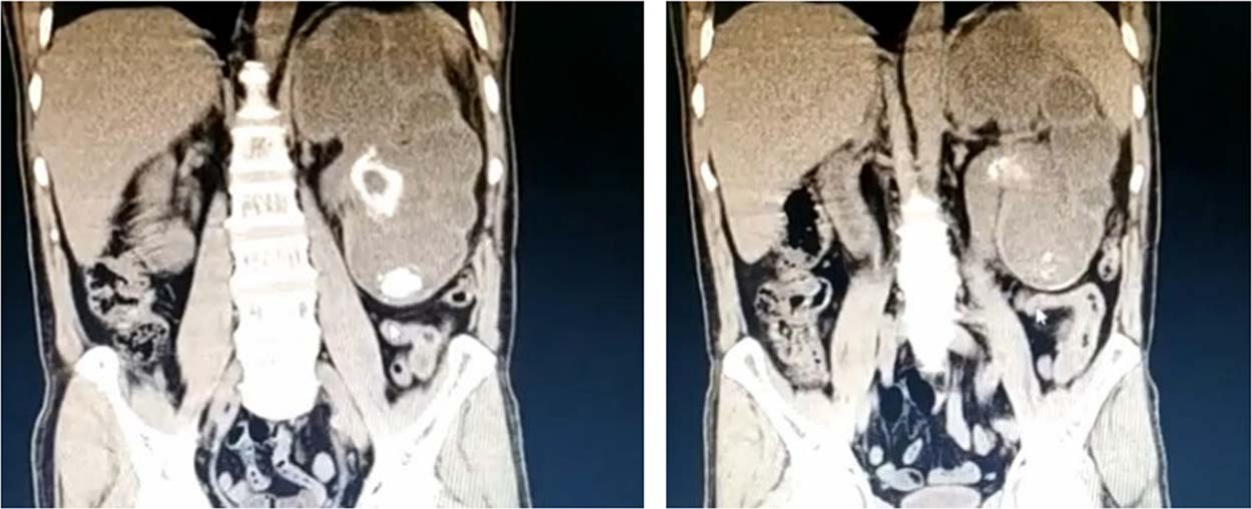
A noncontrast computed tomography sagittal view of the abdomen showing the calculus in the renal pelvis and other obstructing calculi.
